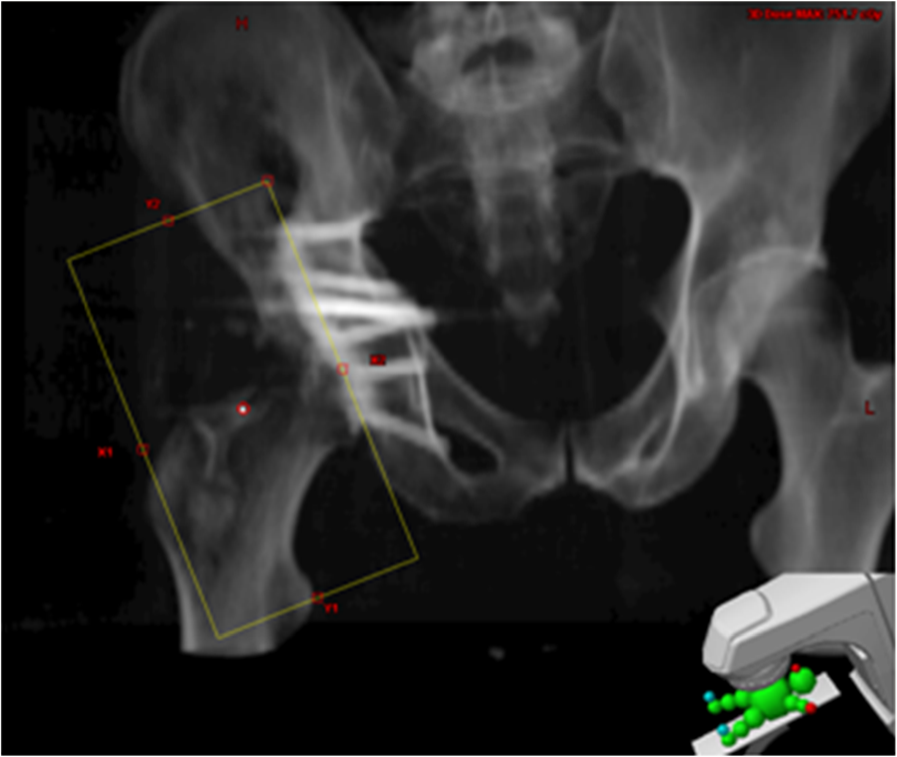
Digitally reconstructed radiograph (DRR) based on original portal images. Portal images from patient’s original treatment were recreated and superimposed on CT scan obtained following the diagnosis of osteosarcoma. An open 8 × 15 field was utilized for patient. Treatment was delivered using 6 MV photons, with a source to skin distance (SSD) or 91.5 cm.
